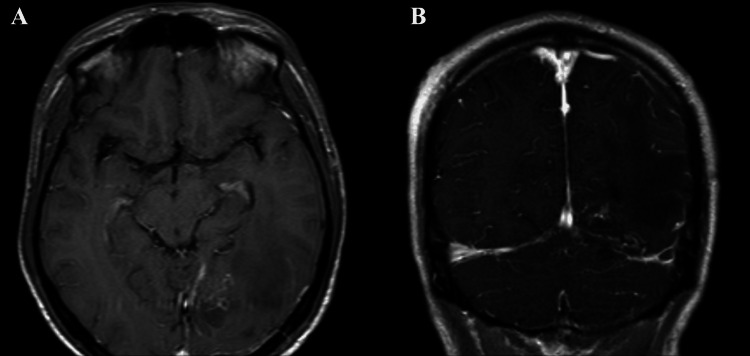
Postoperative brain MRI. Enhanced T1WI in axial (A) and coronal (B) showing the tumor was totally resected.
